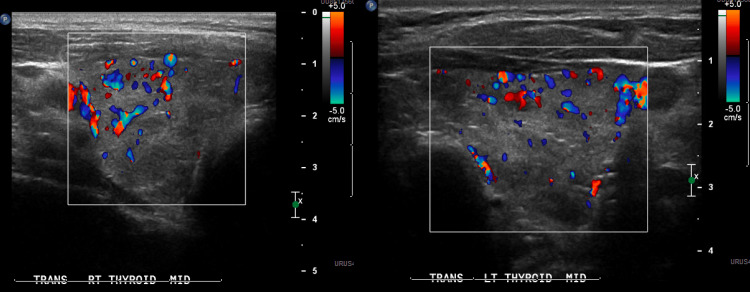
Thyroid ultrasound with color Doppler showing increased blood flow.
